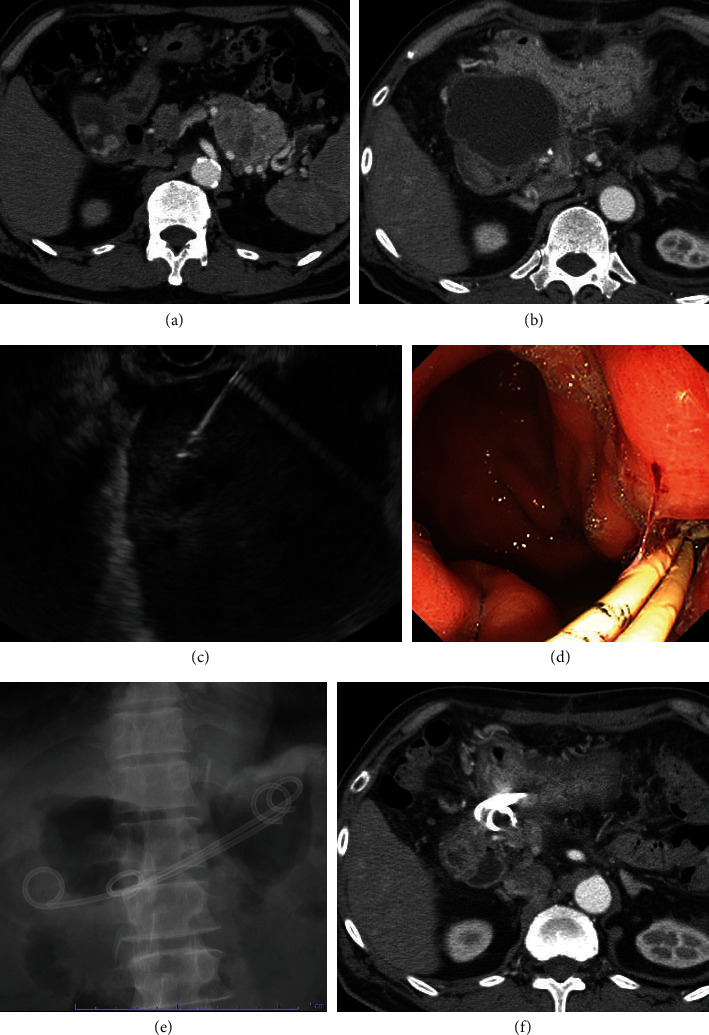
Clinical images of a representative patient who underwent endoscopic ultrasound-guided transluminal drainage (EUS-TD) for postoperative pancreatic fistula (POPF). (a) Preoperative computed tomography (CT) scan reveals a tumor in the pancreatic tail, diagnosed as a pancreatic neuroendocrine tumor. The patient underwent distal pancreatectomy. (b) CT scan performed 14 days after surgery shows an infected POPF of 75 mm diameter. (c) EUS imaging performed on day 15 after surgery shows peripancreatic fluid collection, into which a 19G needle is inserted via a transgastric route. (d) and (e) Two double-pigtailed plastic stents were inserted and can be observed on endoscopic imaging (d) and under fluoroscopic (e) guidance. (f) CT scan performed 2 weeks after EUS-TD reveals resolution of the POPF.
